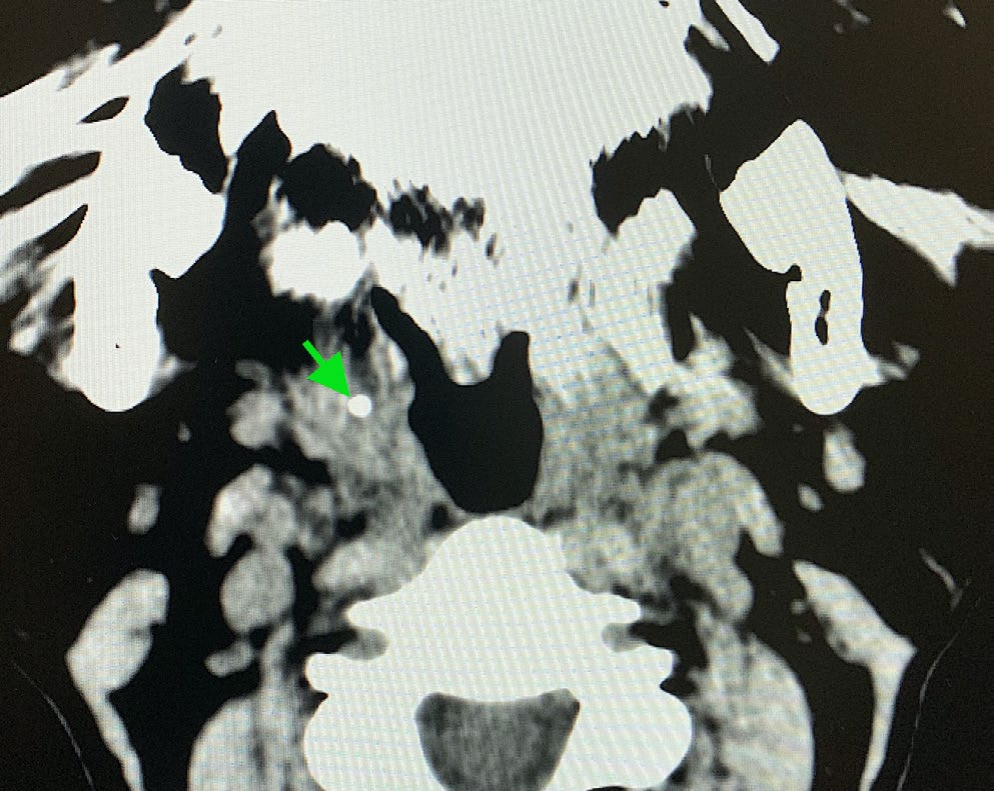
Computed tomography revealed a dense calcified mass in the right palatine tonsil (arrow)
